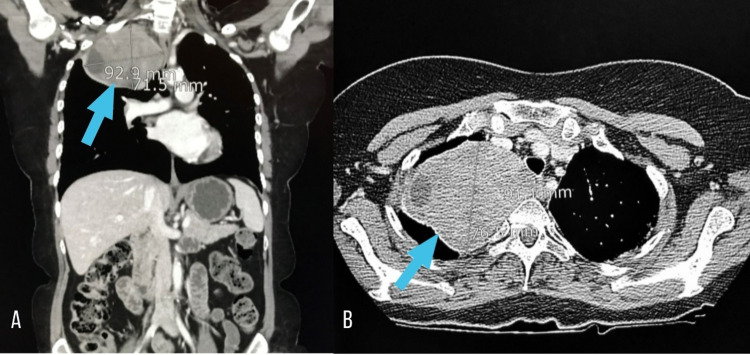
CT scan – Right paraspinal, apical mass with internal cystic areas, approximately 9.5 x 7cm, seen in coronal (A) and axial (B) views, respectively
